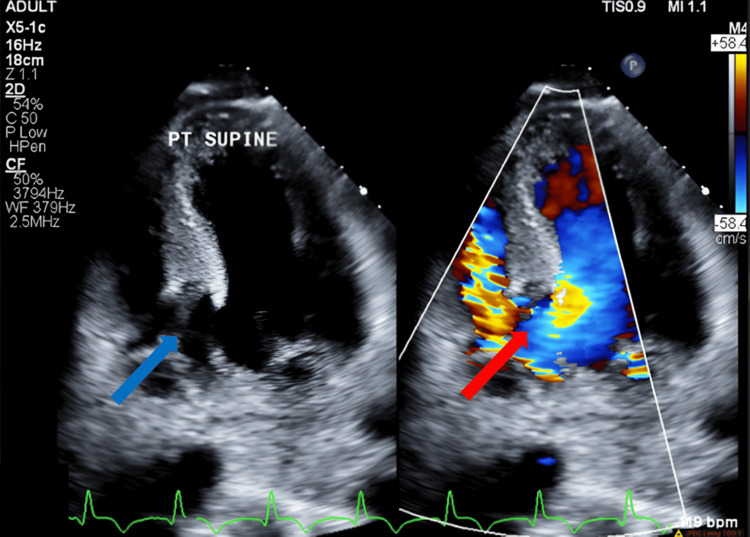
Apical four-chamber view, demonstrating the presence of muscular VSD (blue arrow) with Doppler demonstrating left-to-right shunting (red arrow). VSD: Ventricular septal defect
